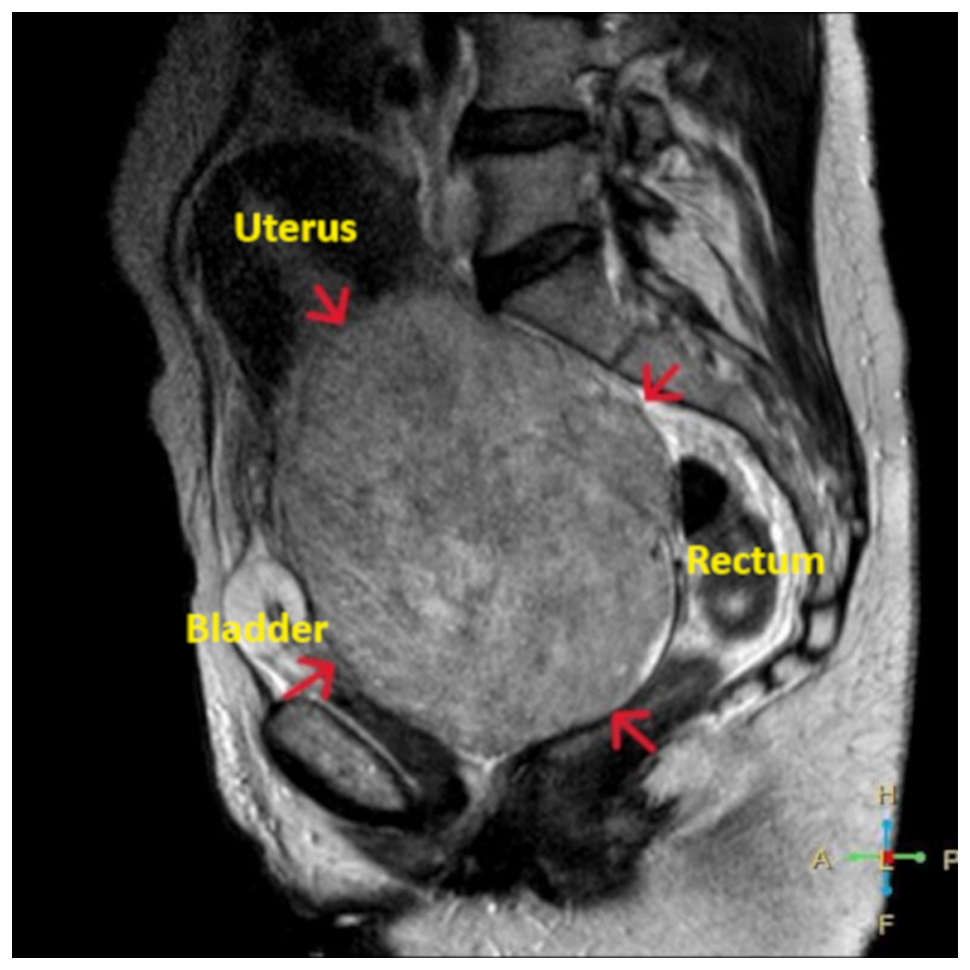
MRI imaging of a well-defined pelvic mass (red arrows) originating from the endocervix corresponding to a giant endocervical fibroid.
